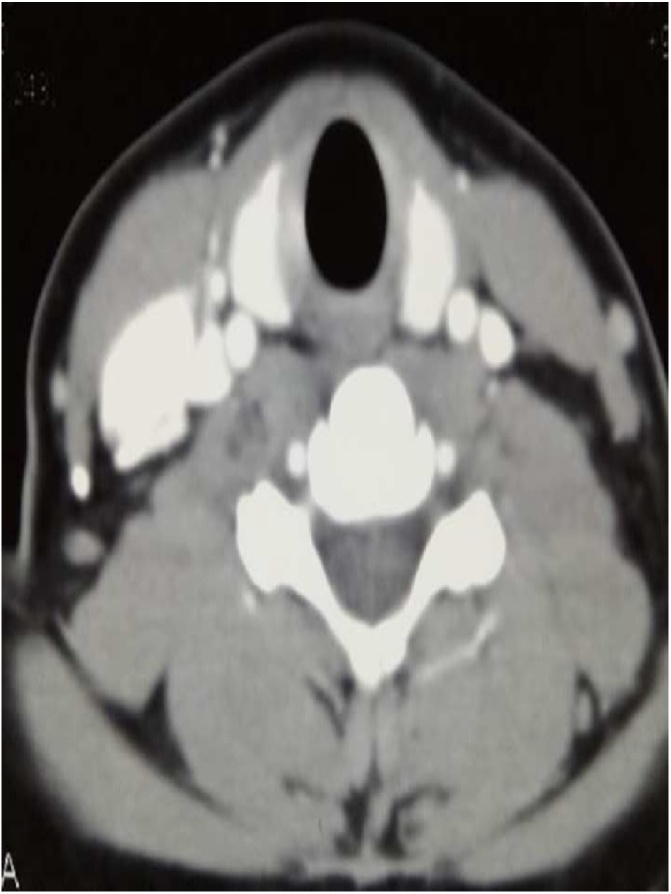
Axial enhanced CT neck showing abnormal enhanced channels on right side appear to be vascular in nature.
